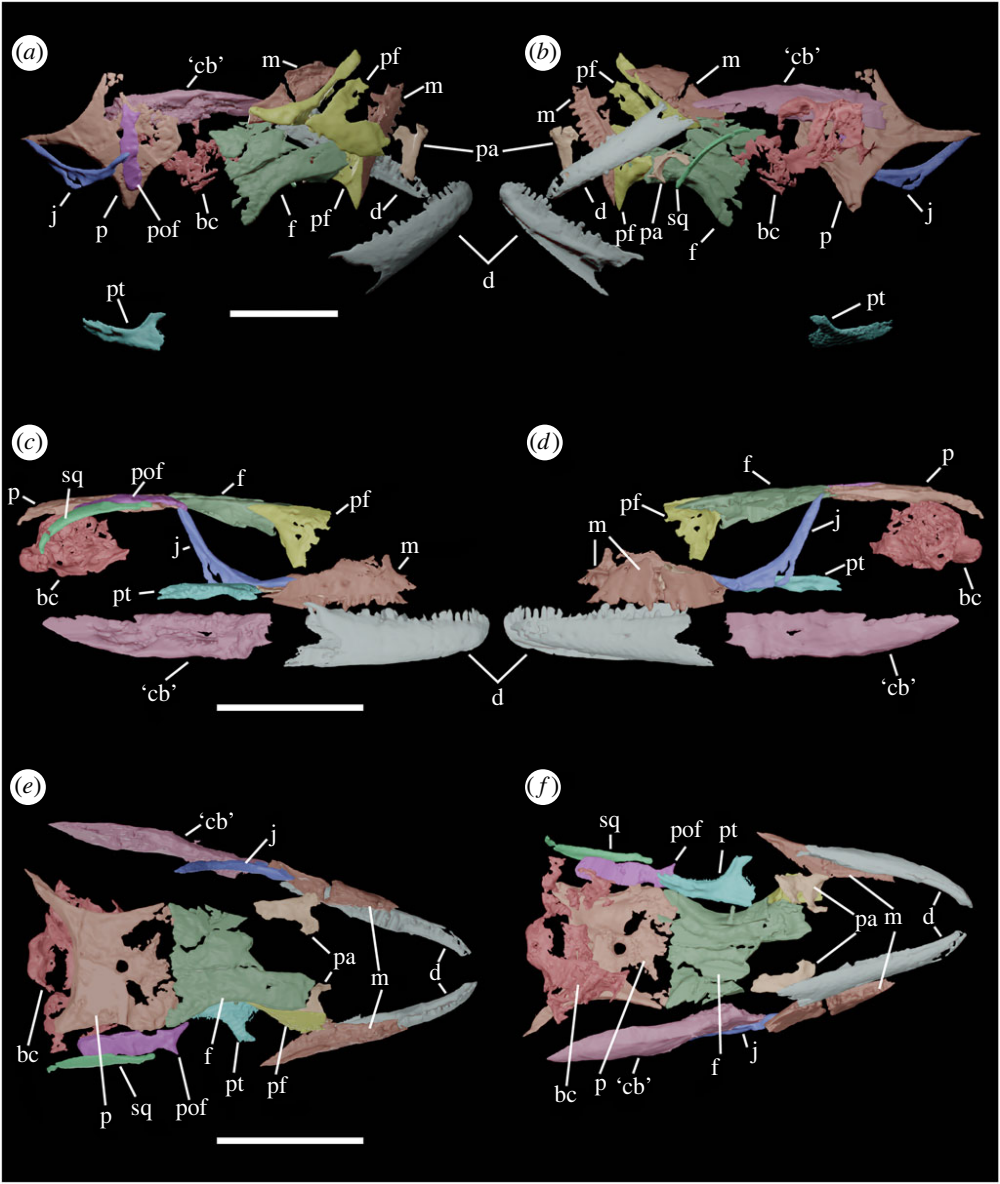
CT Imagery of the skull of *Helioscopos dickersonae* (DINO 15914). (*a,b*) *In situ* skull in dorsal (*a*) and ventral (*b*) views. (*c–f*) Reconstructed skull in left lateral (*c*), right lateral (*d*), dorsal (*e*) and ventral (*f*) views. All scale bars 5 mm. bc, braincase; ‘cb’, fused postdentary elements (compound bone); d, dentary; f, frontal; j, jugal; m, maxilla; p, parietal; pa, palatine; pf, prefrontal; pof, postorbitofrontal; pt, pterygoid; sq, squamosal.
